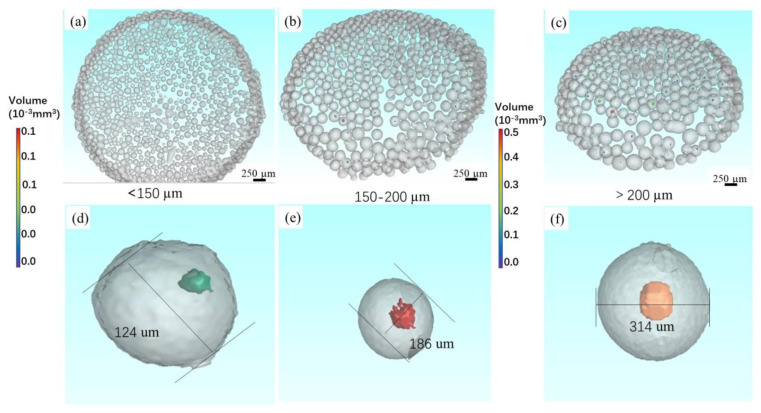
The 3D reconstructed CT of Ti-6Al-4V powders at 8000 RPM with different size ranges. (a) <150 µm, (b) 150–200 µm, (c) >200 µm and the typical pore morphology for particle in the particle range of (d) <150 µm, (e) 150–200 µm and (f) >200 µm.
